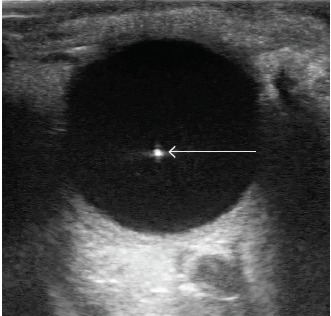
B-mode sonogram showing an echogenic particle (arrow) floating within the body of the vitreous in a patient with asteroid hyalosis.
